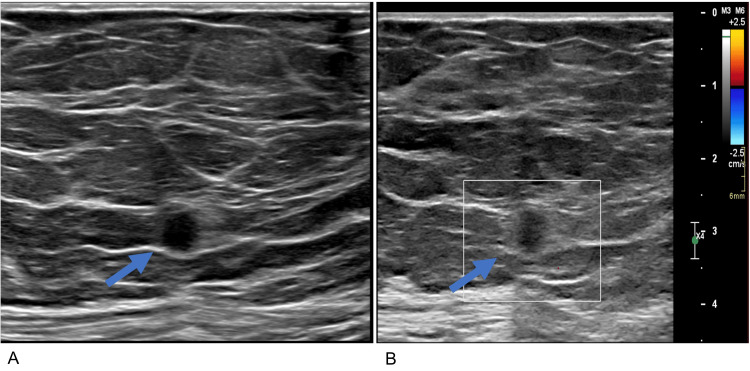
Ultrasound of the right breast Ultrasound imaging of the upper outer quadrant right breast grayscale (A) and color Doppler (B) images of the 9:00 axis 5 cm from the nipple show a round, indistinct, hypoechoic mass (blue arrow) without internal Doppler signal measuring 0.8 x 0.7 x 0.5 cm, which corresponds to the mammographic mass.
